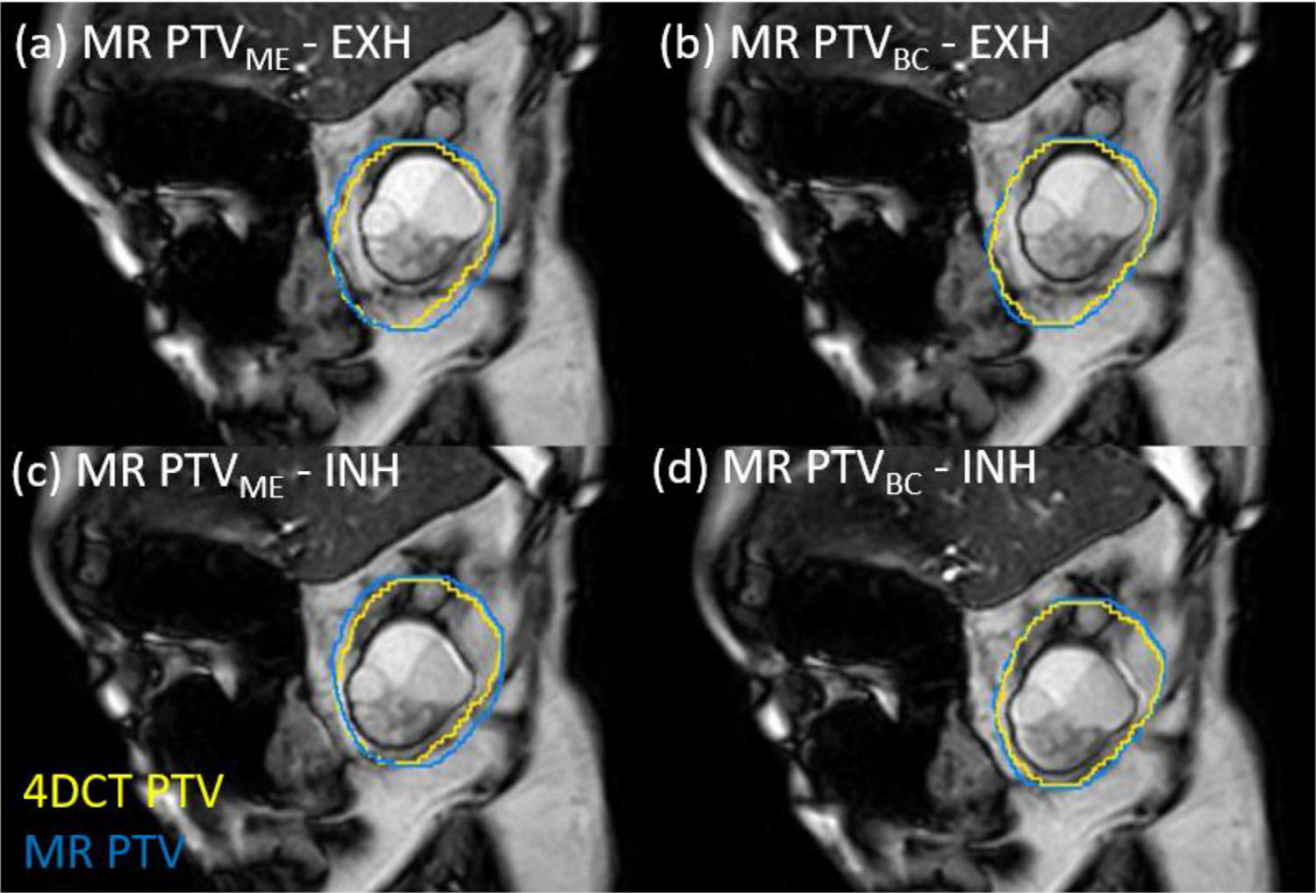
Comparison of patient PTVs with a 5 mm margin created with 4DCT (PTV_4DCT_, yellow), the MR exhale method (PTV_ME_, blue, left) and cine motion method (PTV_BC_, blue, right), on the patient MR for case #1, imaged on the Ingenia MRI. (a) and (c) show the MR PTV_ME_ created with the margin expansion method compared to exhale and inhale cine image, respectively. (b) and (d) show the MR PTV_BC_ created with the Boolean combine method compared to exhale and inhale cine image respectively. MR, magnetic resonance.
